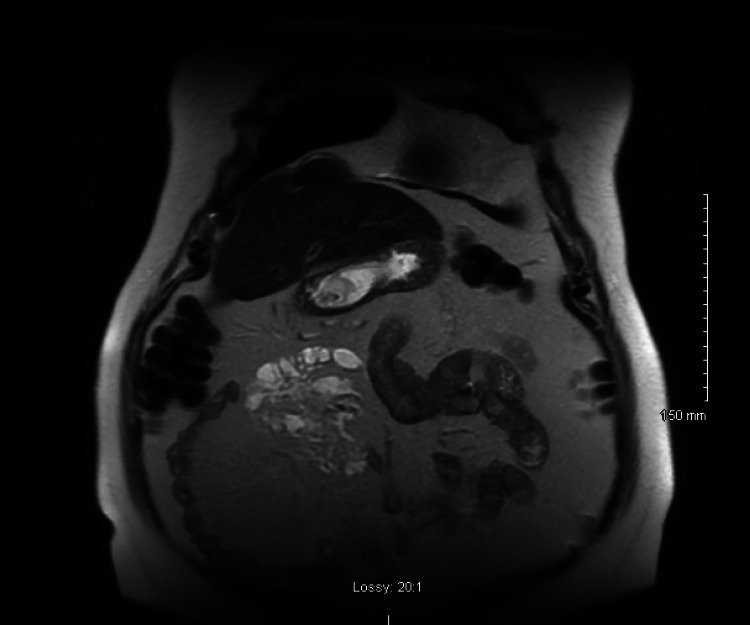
Coronal image of T2-weighted MRI of right upper quadrant lymphangioma with multiple loculations.
